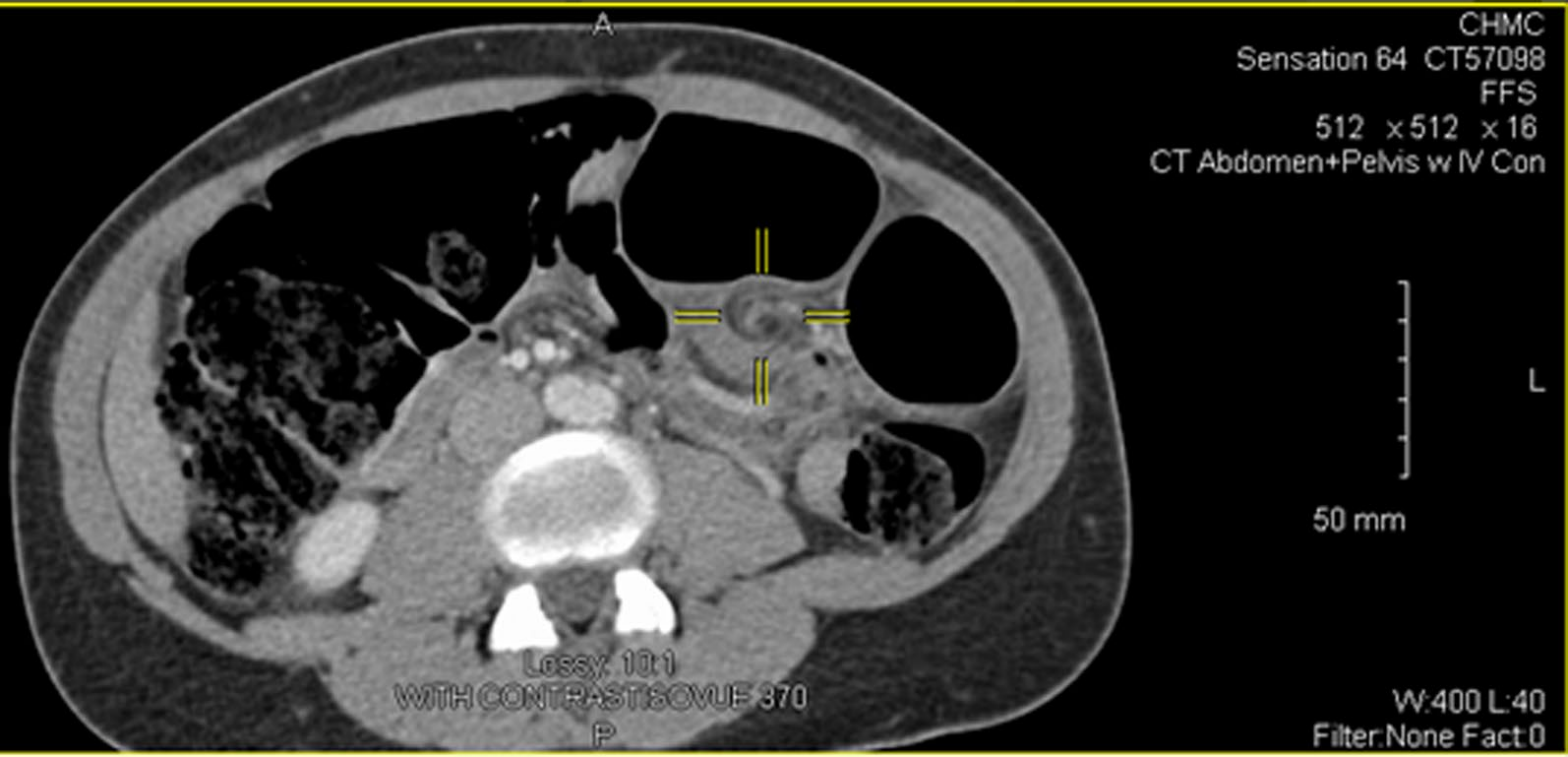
Axial CT showing “whirl” sign (emphasized by the yellow cursor) and distended large bowel
